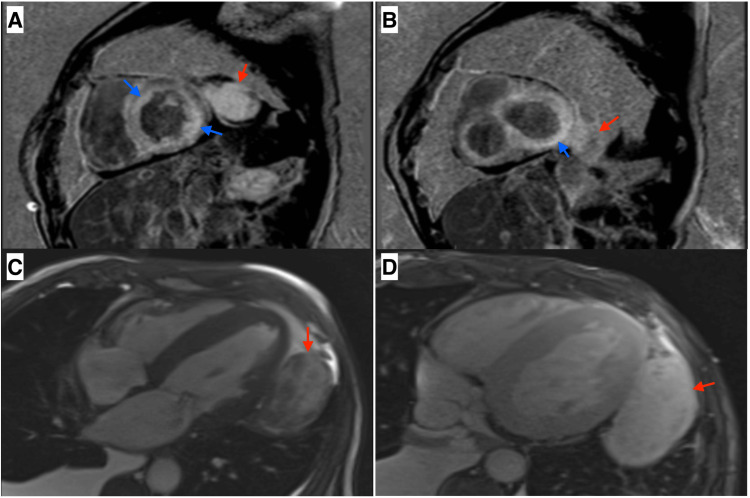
Cardiac MRI. Short axis view of the (A) basal ventricular and (B) mid-heart segments demonstrating with blue arrows global patchy diffuse mid-wall delayed enhancement, patchy infiltrate, and subendocardial distribution of the enhancement, typical of amyloidosis. Mediastinal mass delineated by red arrows showing (C) heterogeneity and (D) gadolinium-enhanced hyperintensity on T2.
